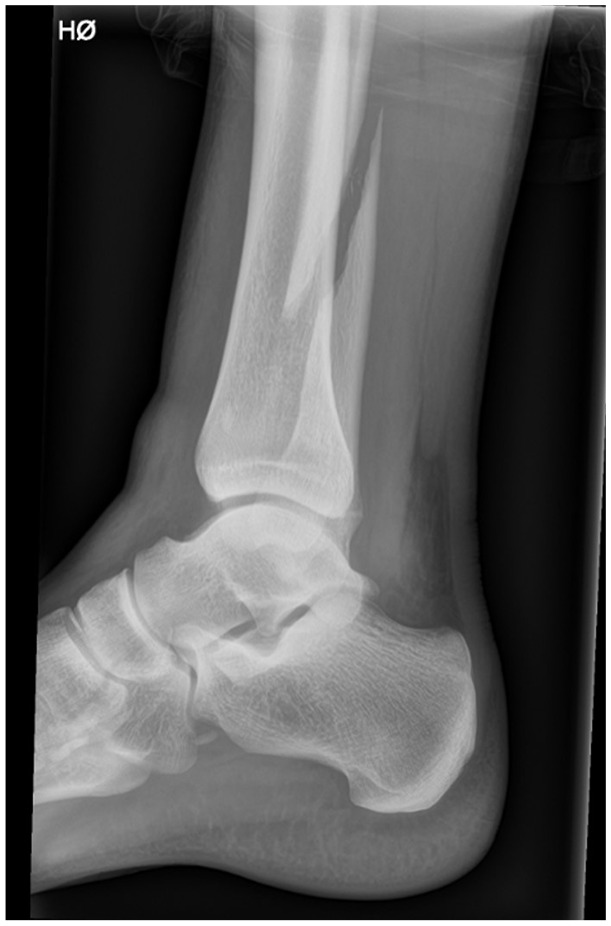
Sagittal plane radiograph showing a suprasyndesmotic ankle fracture.
